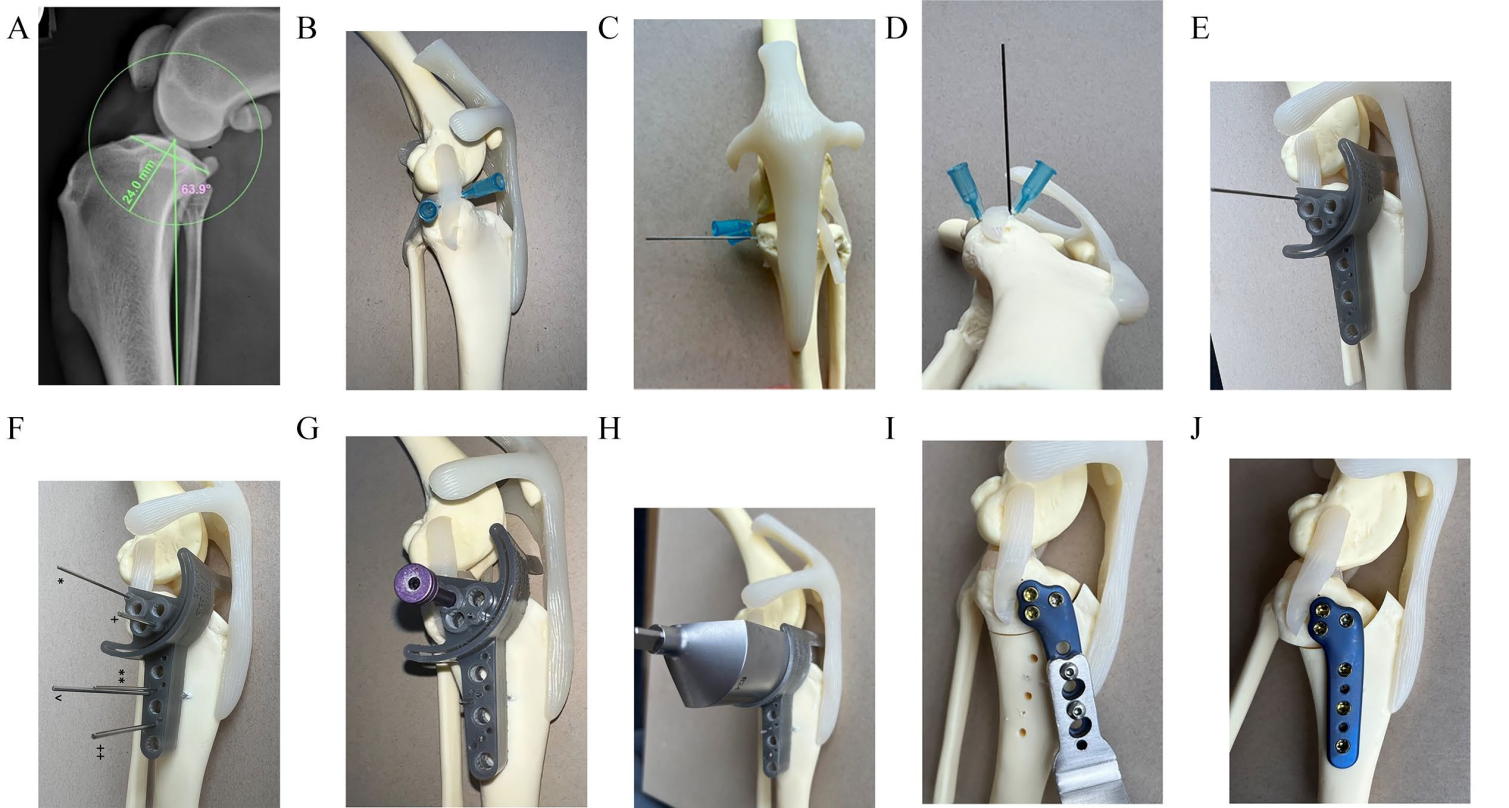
ProCut TPLO surgical technique. (A) Preoperative, calibrated radiographs are taken to determine tibial plateau angle (TPA) and p-TPLO template size. D1/D2 measurements are not required. (B) Step 1: Stifle joint surface is delineated with hypodermic needles after making standard medial approach to the proximal tibia. (C) Step 2: The 1.0 mm k-wire [proximal centering pin (PCP)] is placed 2–3 mm distal to the joint surface, approximately 3 mm into the proximal tibia just cranial to the medial collateral ligament. The PCP represents desired osteotomy trajectory and is assessed in the frontal and (D) coronal plane. Trajectory can be modified if needed by bending the PCP at its junction with the tibial cortex. (E) Step 3: Position the p-TPLO template on the PCP with the patellar tendon shield caudal to the patellar tendon. (F) Step 4: Once the template is positioned on the PCP (*), a second 1.0 mm k-wire (distal centering pin [DCP]) () is placed to center the distal aspect of the template and trajectory is again assessed. 1.6 mm proximal (^+^) and distal (^++^) temporary fixation pins are then placed, followed by a non-parallel fixation pin (^) to fix the desired trajectory. (G) Step 5: Using the p-TPLO drill sleeve in the template drill holes, drill all six screw holes using 3.0 mm bit. (H) Step 6: Execute radial osteotomy via the saw blade slot. (I) Step 7: The template, PCP, DCP, and distal pins are removed. During proximal screw insertion, the bone plate with rotation handle attached was manually held over the piloted screw holes, and all proximal Morse taper locking screws were inserted until initial contact with the bone plate, then sequentially tightened, pulling the plate flush to the bone, facilitating compression. (J) Step 8: Rotate the proximal bone segment until the piloted distal screw holes are aligned with the holes of the bone plate, then insert and tighten distal screws and detach rotation handle.
